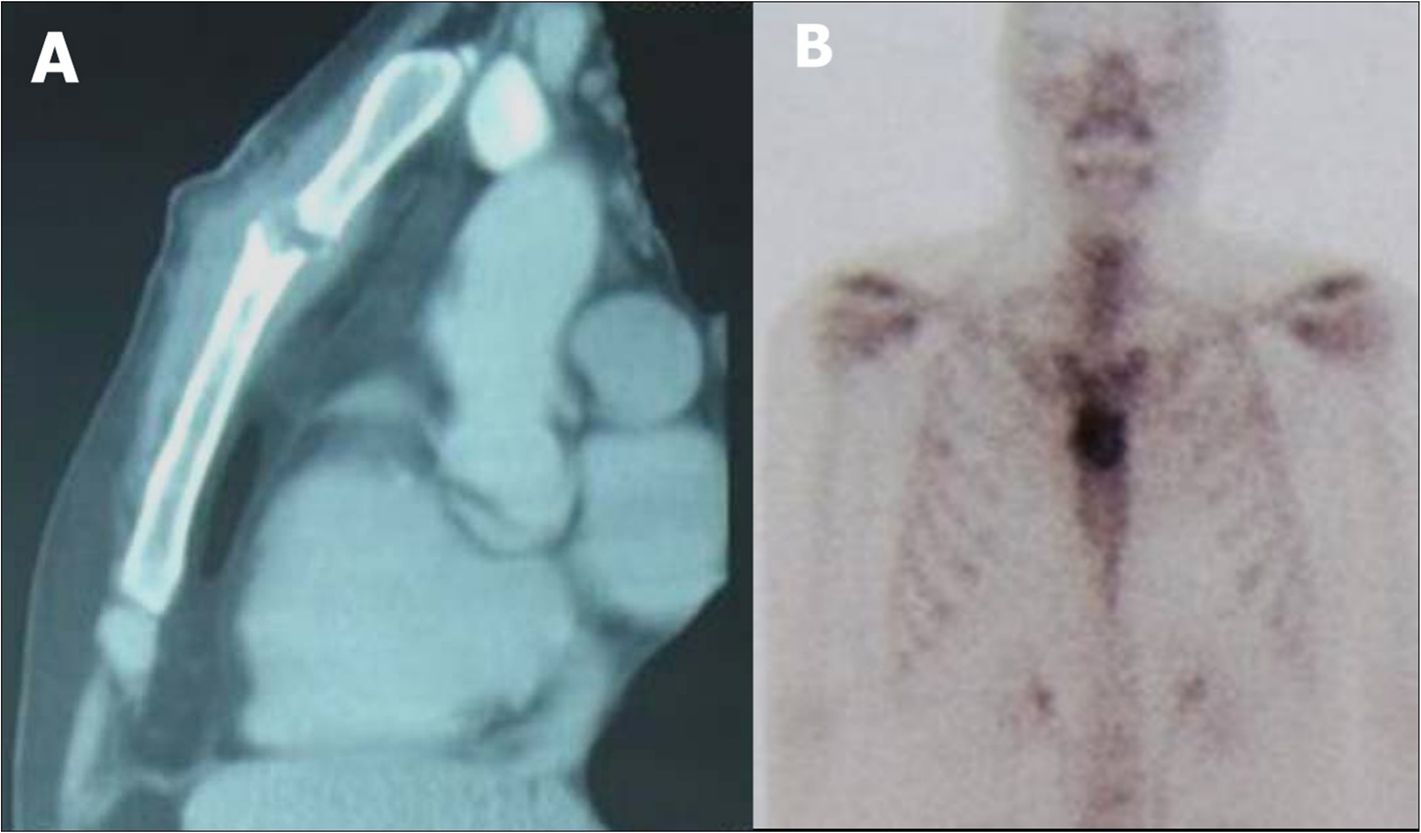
Day 30 postoperative. A: Chest computed tomography showing involution of bone injury. B: Bone scintigram showing moderate increase in osteoblastic activity in the sternum associated with moderate-to-marked increase in capillary permeability, suggesting bone remodeling and absence of osteomyelitis.
